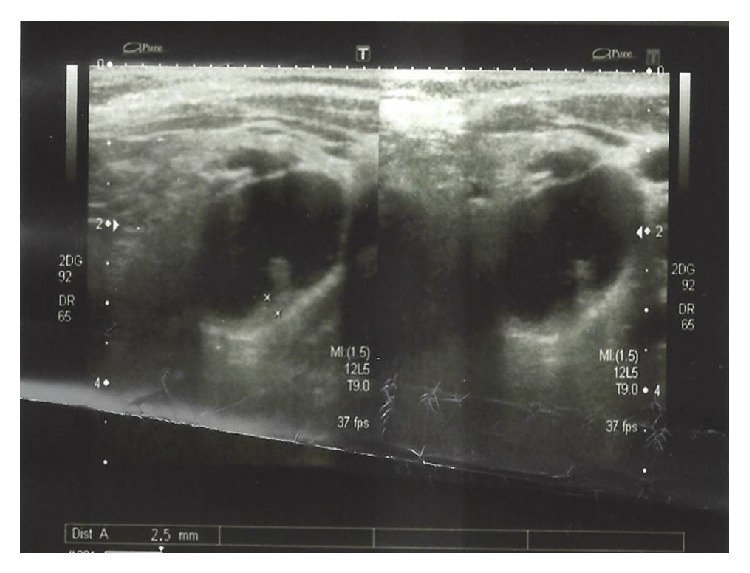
Ultrasonographic image of cystic lesion (17 × 26 mm) in left lobe of thyroid gland.
